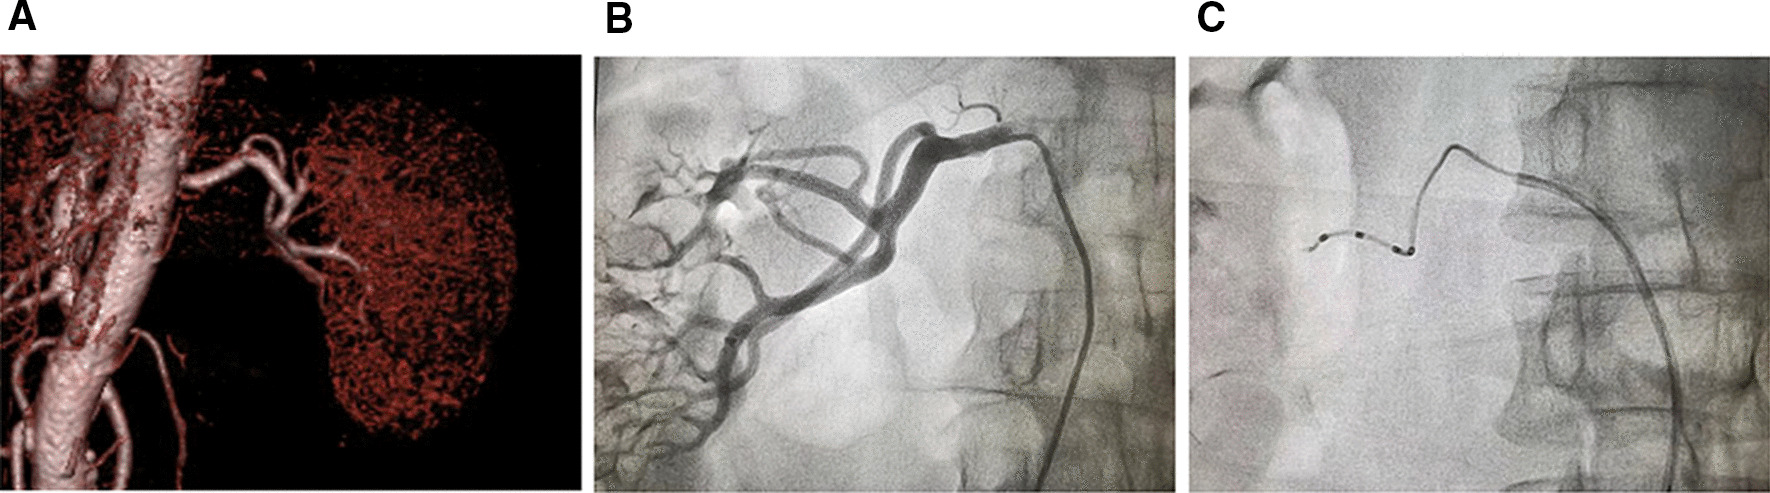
Angiography and denervation of the right renal artery. a Three-dimensional reconstruction of computed tomography angiography showing the right renal artery. b Angiography of the right renal artery. c Denervation of the right renal artery using an Iberis ablation catheter with a regular guiding catheter
